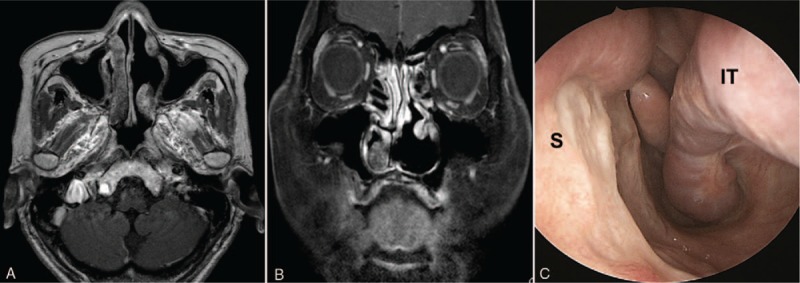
Follow-up paranasal sinus imaging and nasoendoscopic view at 18 months after surgery. (A) Axial and (B) coronal magnetic resonance imaging of the paranasal sinuses show a clear nasal cavity without recurrence. (C) Nasoendoscopy reveals complete resolution of the tumor and recovery of the septal mucosa. IT = inferior turbinate, S = septum.
